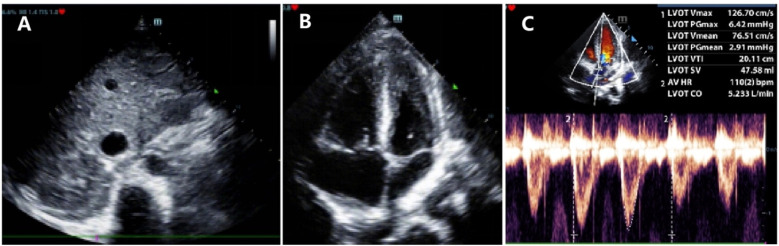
Point-of-care ultrasound of the heart. (A) Dilation of the inferior vena cava. (B) Right heart enlargement with increased wall motion. (C) Left Ventricular Output of 5.2 L/min.
